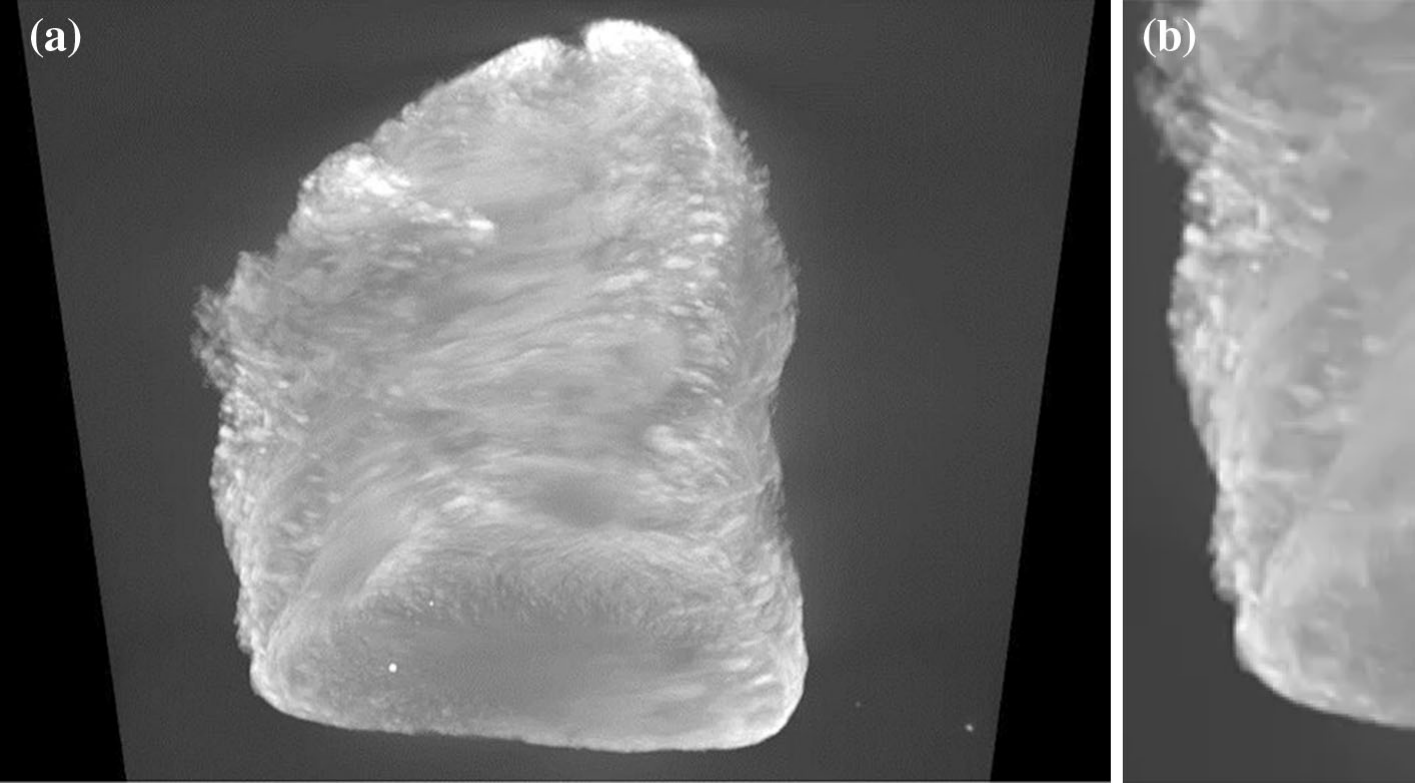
Computerised X-ray Tomography (CXT) image of a a monolithic fragment following mercury entrapment, and b a close-up view of the left limb of the monolith image. The* bright white* ovoid regions correspond to mercury-filled bubble pores
